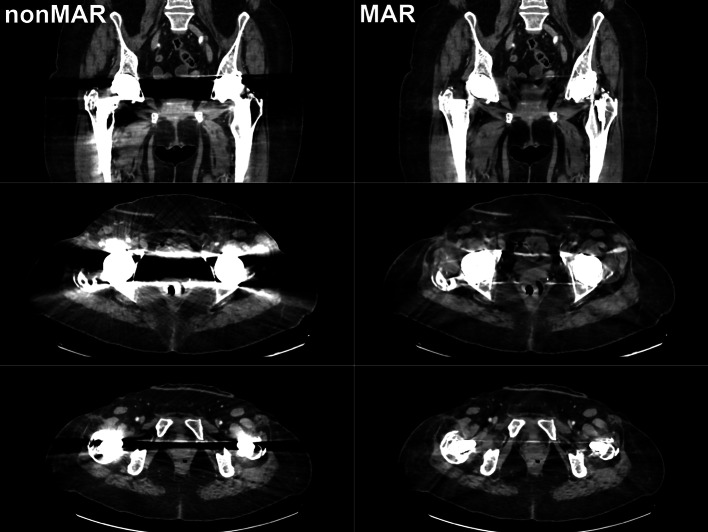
CT images with and without MAR in a patient with bilateral hip prostheses. Selected coronal (upper row) and axial (second and third row) slices are shown from CT reconstructed without MAR (left column) and CT reconstructed with MAR (right column)
